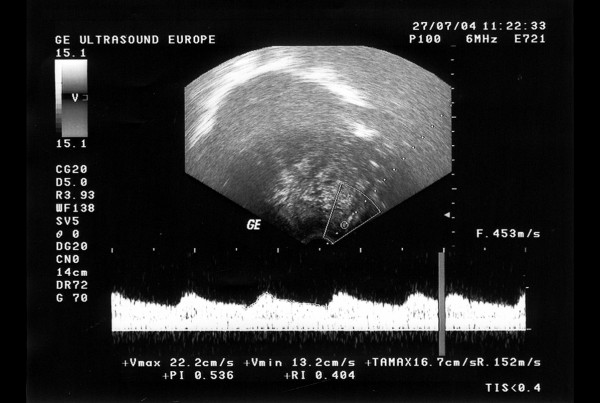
Typical Doppler image obtained from a uterine myoma.
